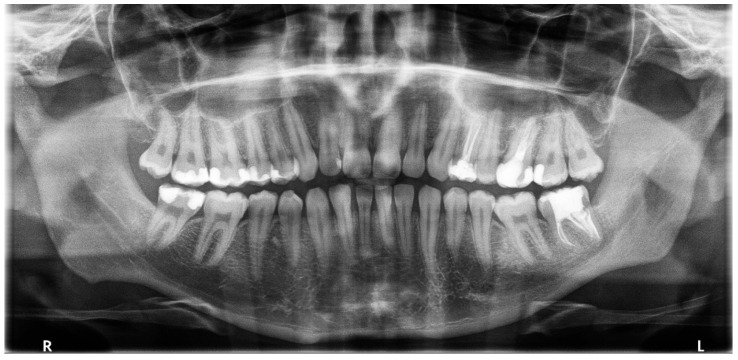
Six-month post-operative OPG radiograph after root canal treatment (fifth appointment).
